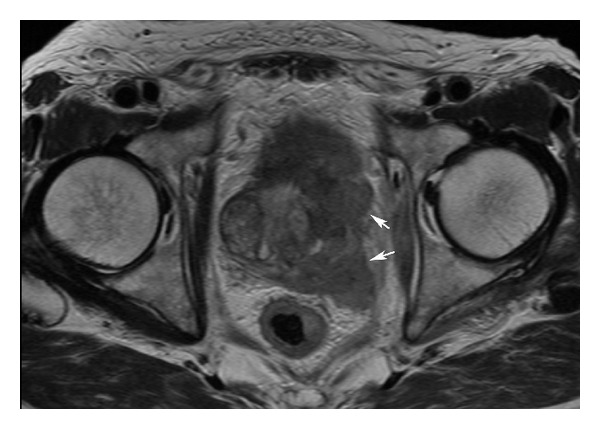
T2-weighted MR imaging demonstrated a tumor originating from the left peripheral zone of the prostate (arrow).
